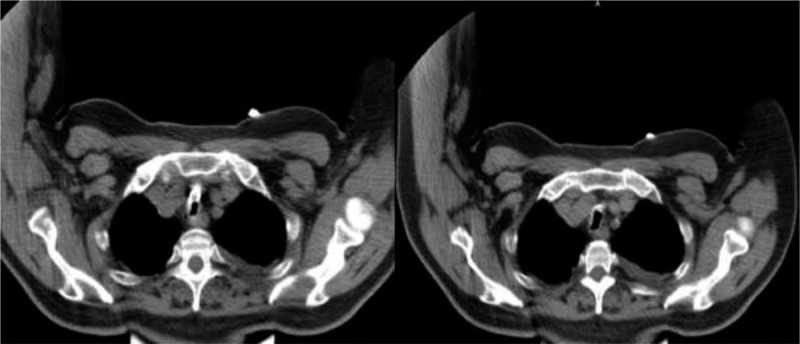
CT scan (mediastinal window) of this 72-year old male patient showed signs of tracheal stenosis, as indicated by the white arrow.
